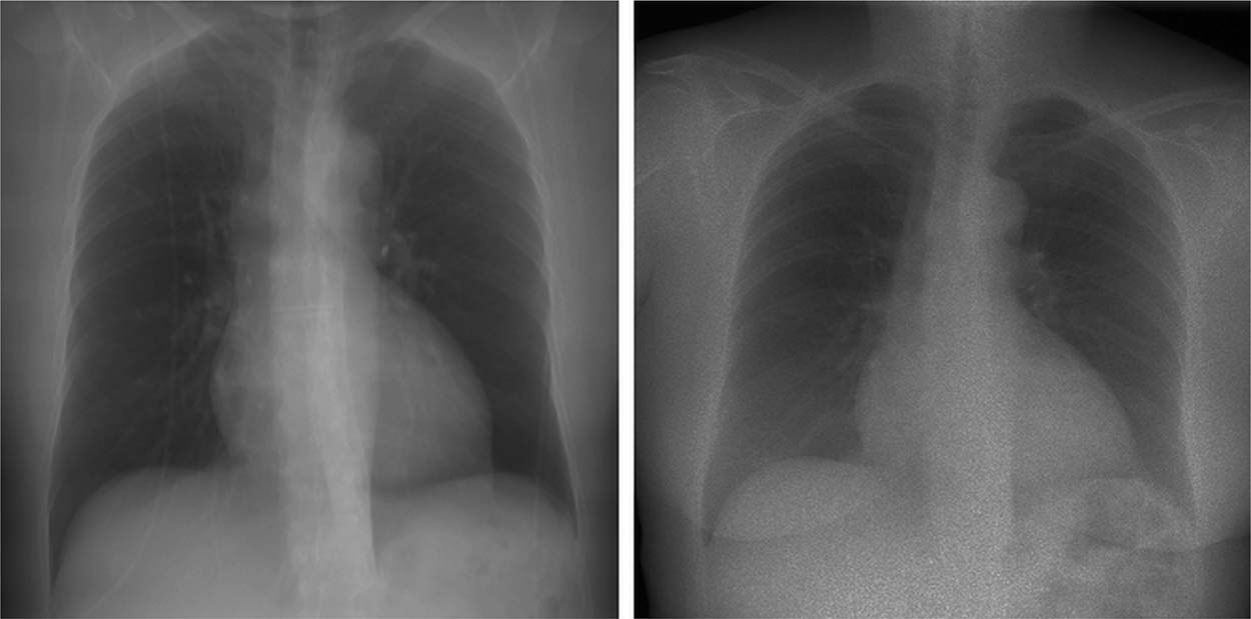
A real projection image (left) and the synthetic projection image generated from the corresponding CT volume (right) showing anatomical misalignment between acquisitions.
